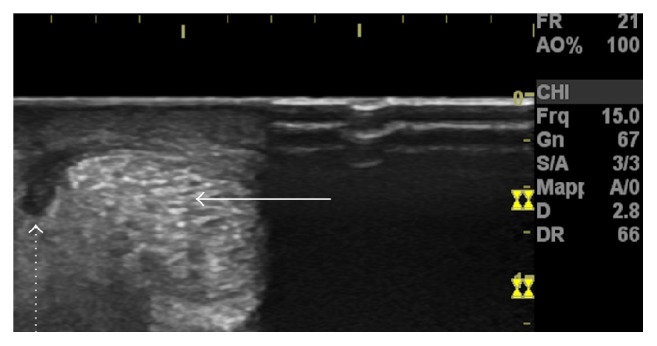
High resolution cross-sectional ultrasound of the Achilles tendon suffering from acute paratendinopathy. Laterally to the body of the Achilles tendon (*filled arrow*), a hypoechoic area is visible (*dotted arrow*), which is compatible with fluid collection between the tendon and the paratenon.
